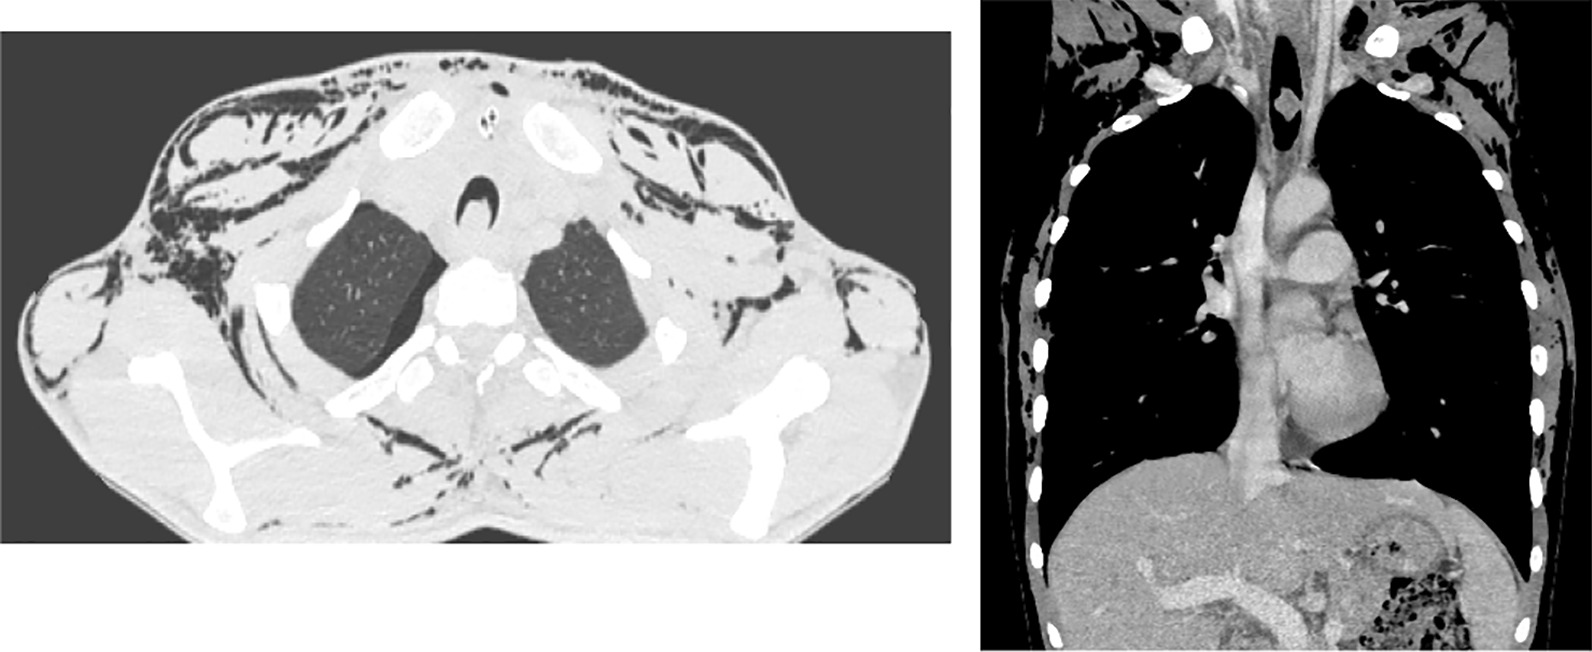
Computed tomography Scan that showed extensive subcutaneous emphysema, intraluminal soft tissue mass arising from the right posterolateral aspect of trachea about 5 cm from the carina with small air collection in the anterior mediastinum, right pleural, and anterior pericardial spaces
